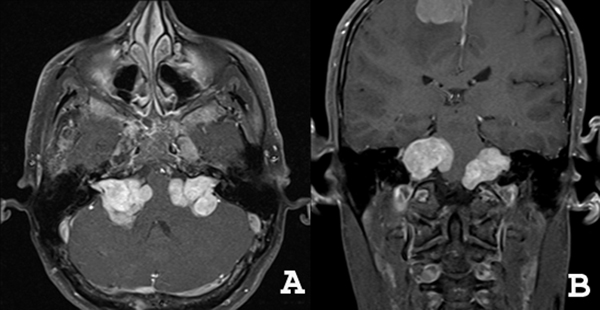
Vestibular schwannomas. Axial (A) and coronal (B) enhanced T1-weighted MR images demonstrating bilateral solid masses in the cerebellopontine angles, compressing the pons and the 4th ventricle. In addition, a right parafalcine meningioma is seen.
